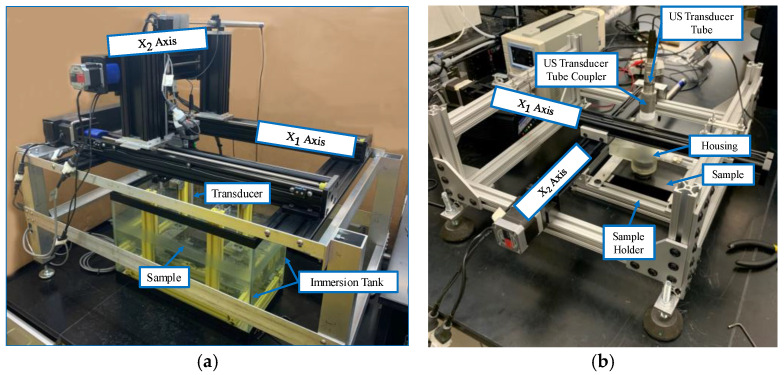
Ultrasonic inspection systems, (a) Representative immersion scanning system, and (b) Out of tank portable housing system.
